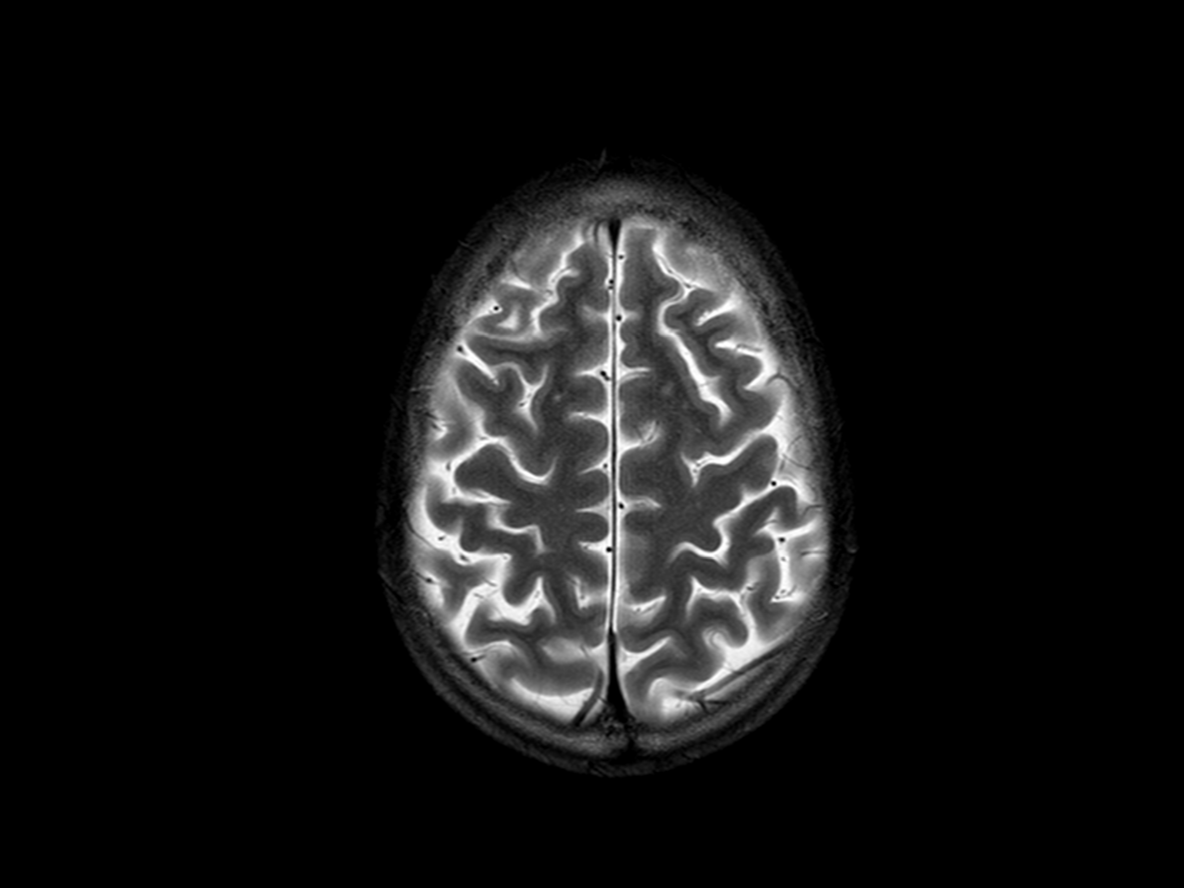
The photo B. submitted of his brain MRI scan as a self-portrait for a photography project entitled “*Looking out to see within*”
